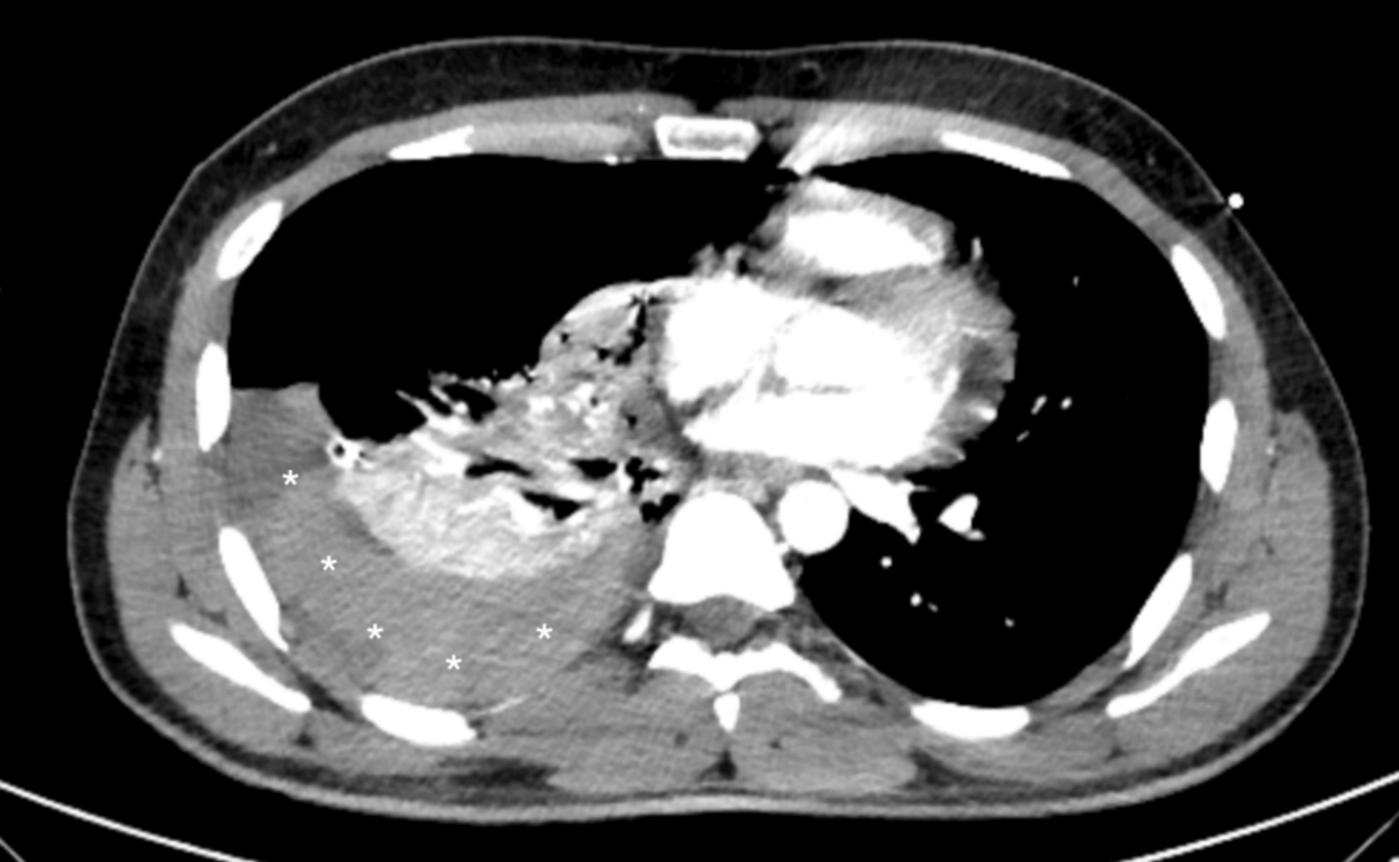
Axial contrast-enhanced chest CT demonstrating a moderate right-sided hemothorax Note: asterisks denote the area of pleural effusion
